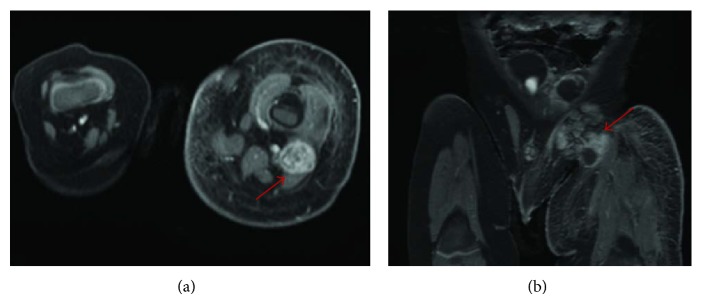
Axial (a) and coronal (b) T1-contrast-enahnced MR images through the thighs are shown. There is a heterogeneously enhancing mass in the posterior thigh involving the adductor compartment (arrow in (a)). There are also several enlarged external iliac lymph nodes: some with cystic and necrotic changes (arrow in (b)). Note the enlargement of the left lower extremity and significant soft-tissue edema and fat stranding.
